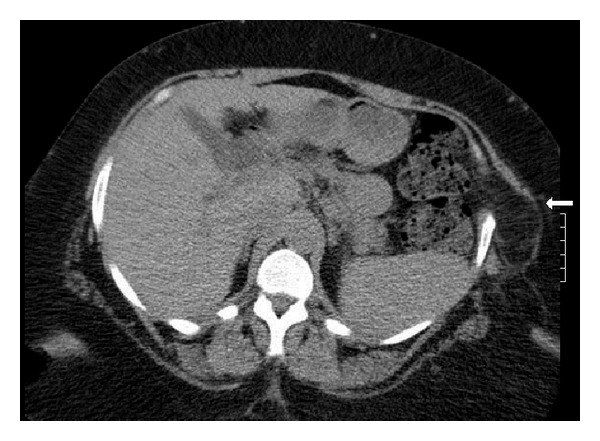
Axial CT view: intercostal hernia between 10th rib and 11th rib at left midaxillary line (white arrow).
